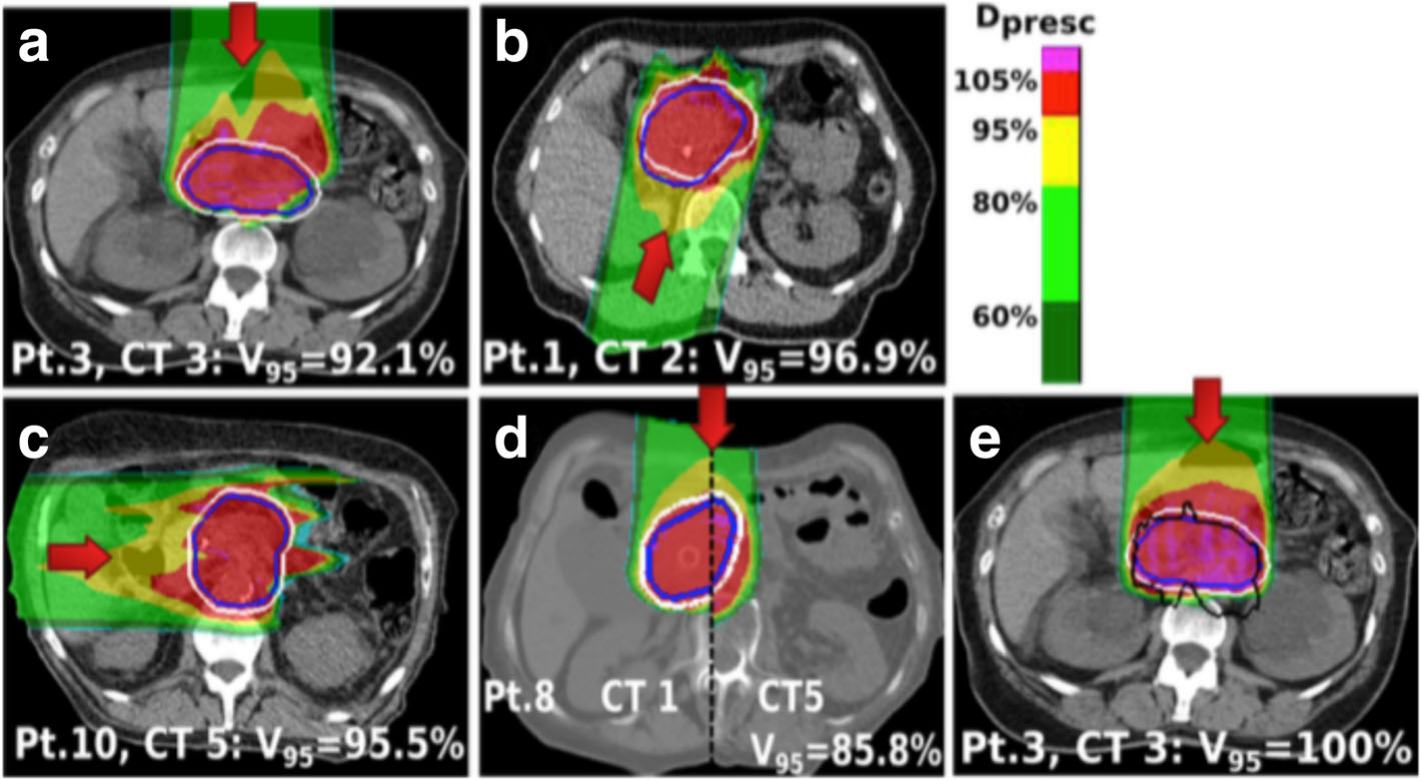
Forward dose distribution of the optimized plan for the ITV_G5_ (*white contour*) in one of the weekly CTs. Different inter-fractional changes are disclosed for analysis of the CTV (*blue*) V95_CTV_: (a) Patient 3, for which the use of an anterior beam results in CTV under-dose due to changes in the bowel/stomach, (b) Patient 6, the oblique posterior beam does not overcome the tumor shift and deformation. c Patient 10, irradiation with a lateral right beam, where the range changes resulted from the bowel and liver position led to health tissues overdose. d Patient 8, an anterior beam is not robust to the accentuated weight loss (visible the comparison of the distribution in the first and last week CT). e Dose distribution for the same CT and patient as in the figure a) but showing the retrospectively determined ITV_WEPL_ (*black contour*) in comparison to the ITV_G5_
